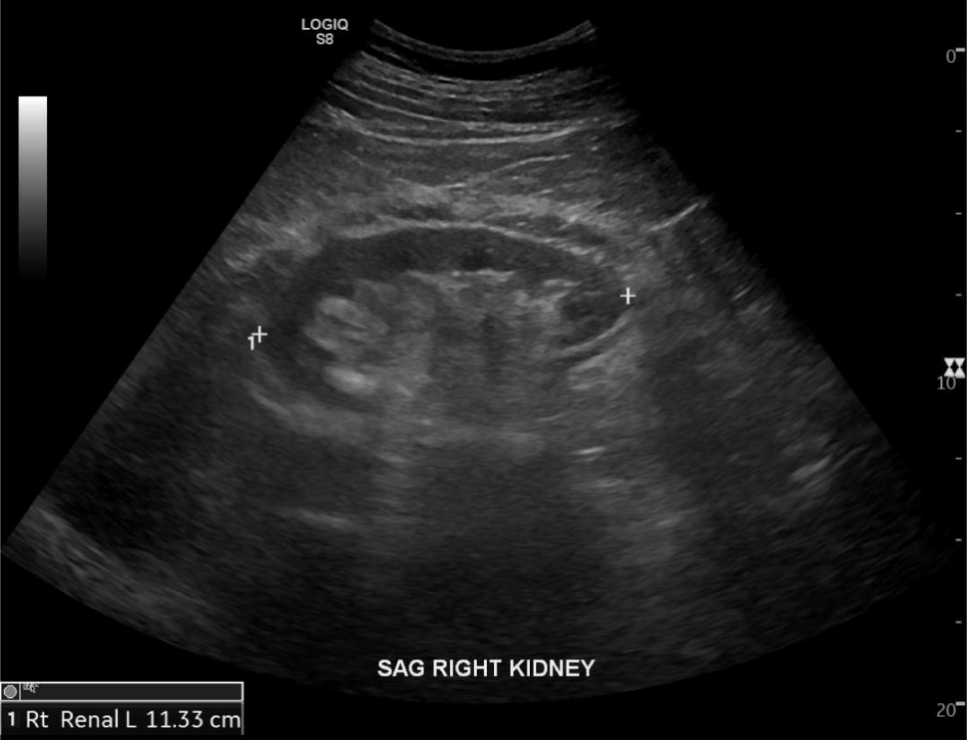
Ultrasound showing diffuse cortical thinning and mildly echogenic renal parenchyma
